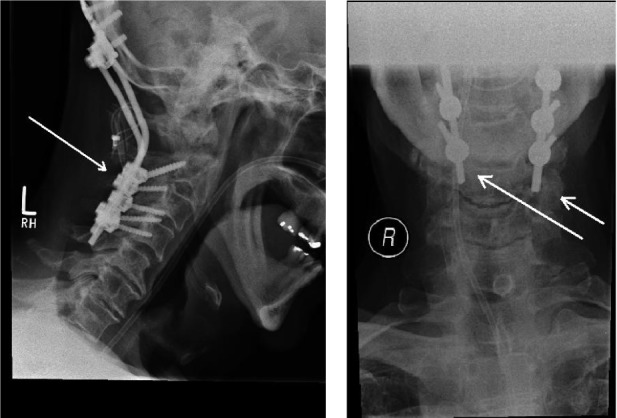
X-rays after spinal fusion
